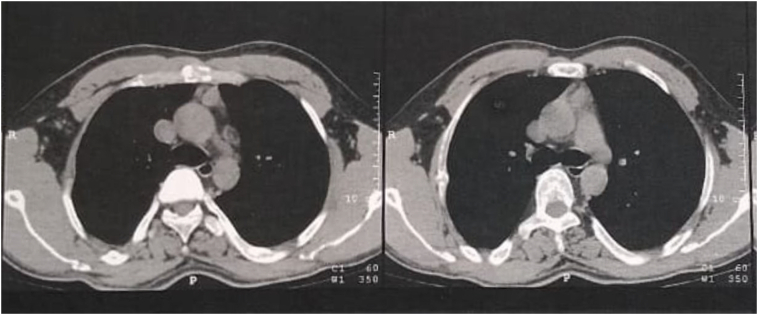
CT chest showing anterior mediastinal mass.
